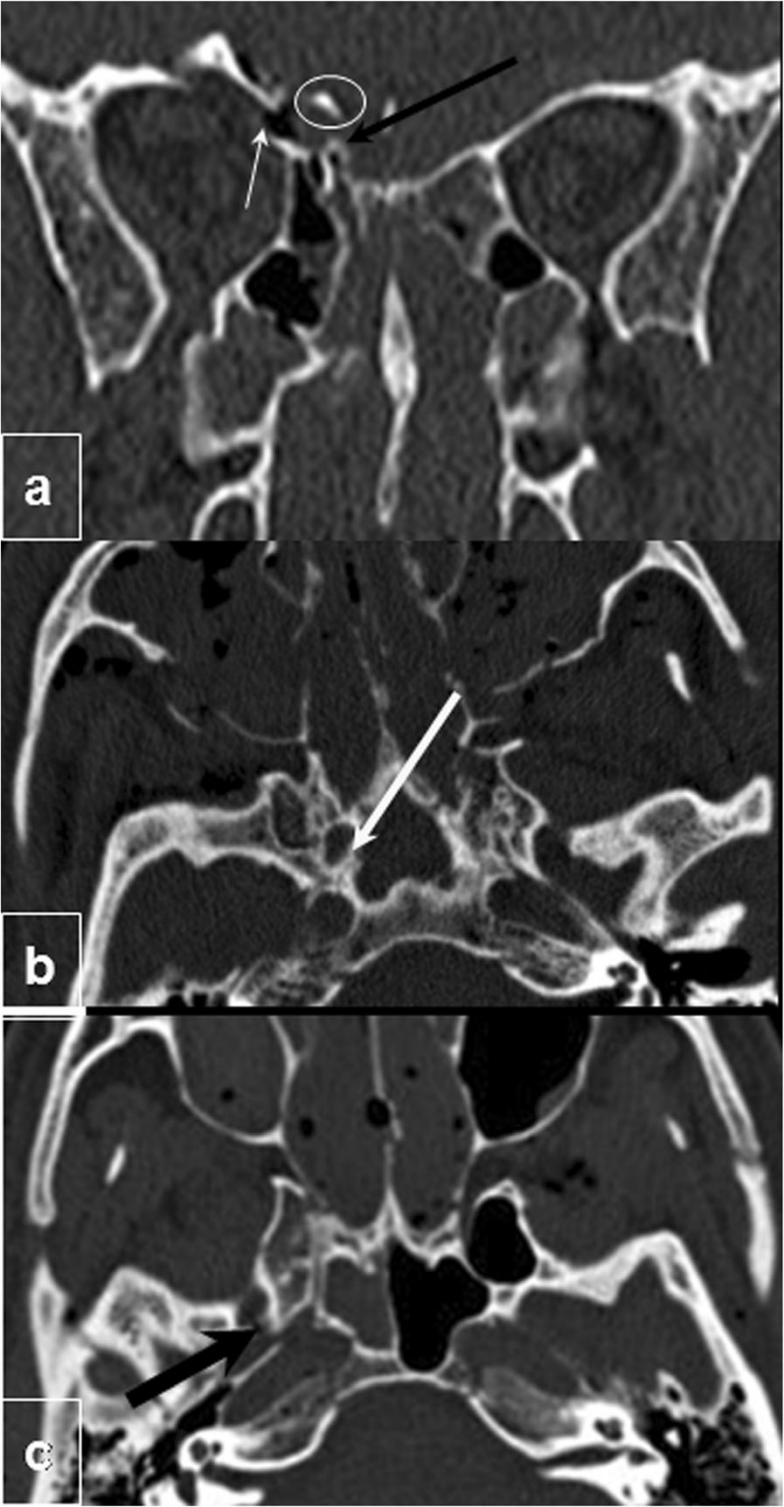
Skull base extension. a Anterior cranial fossa: coronal CT image shows a fracture extending to the lamina cribrosa, communicating the ethmoid cells with the anterior fossa of the cranium on the right side (black arrow) and spanning the orbital roof (white arrow). There is a small intracranial bone fragment (circled). This fracture may cause cerebrospinal fluid rhinorrhea and even infectious intracranial complications. b, c Middle cranial fossa axial CT images from two different patients show extension of the fracture into the sphenoid bone and skull base affecting the carotid canal (white arrow in b) and foramen ovale (black arrow in c) with possible injury to carotid arteries and cranial nerves
